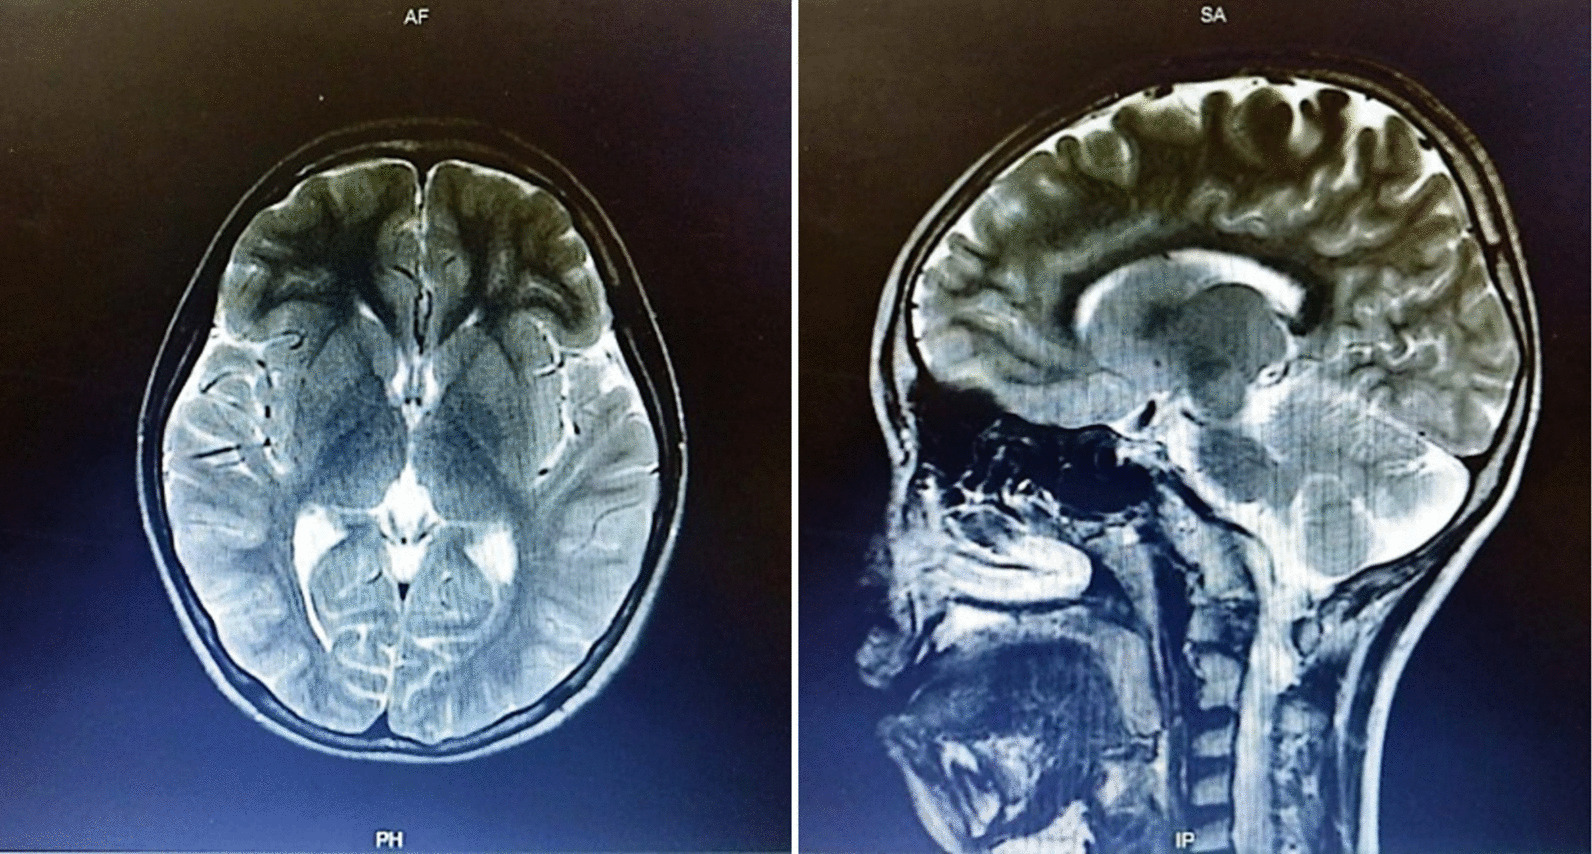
Axial brain magnetic resonance imagery shows cerebral atrophy, bilateral frontal subarachnoid enlargement, bilateral occipital lobe polymicrogyria, and a neuroglial cyst in the right temporal lobe
